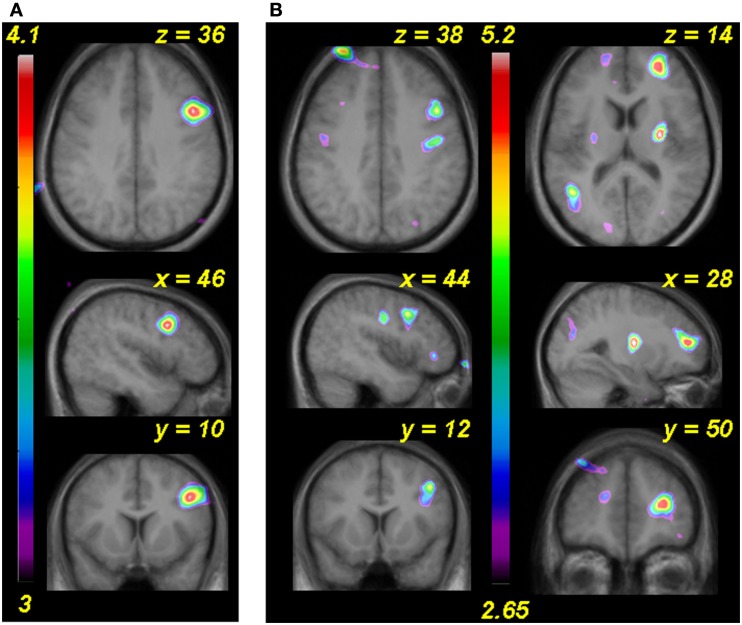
(A) Functional MRI data for the analysis comparing post-training to pre-training global BOLD changes in the passive task versus silence. (B) As in (A) but for active task. In both cases an area of the right dorsolateral frontal cortex shows more activity after training.
